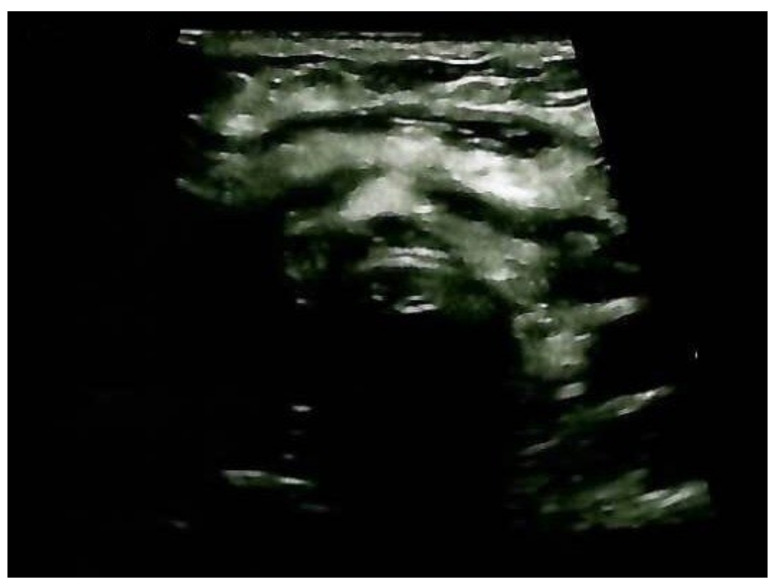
Anterior cervical ultrasonography after total thyroidectomy and ETTM removal: cervical edema and no thyroid tissue remnants.
